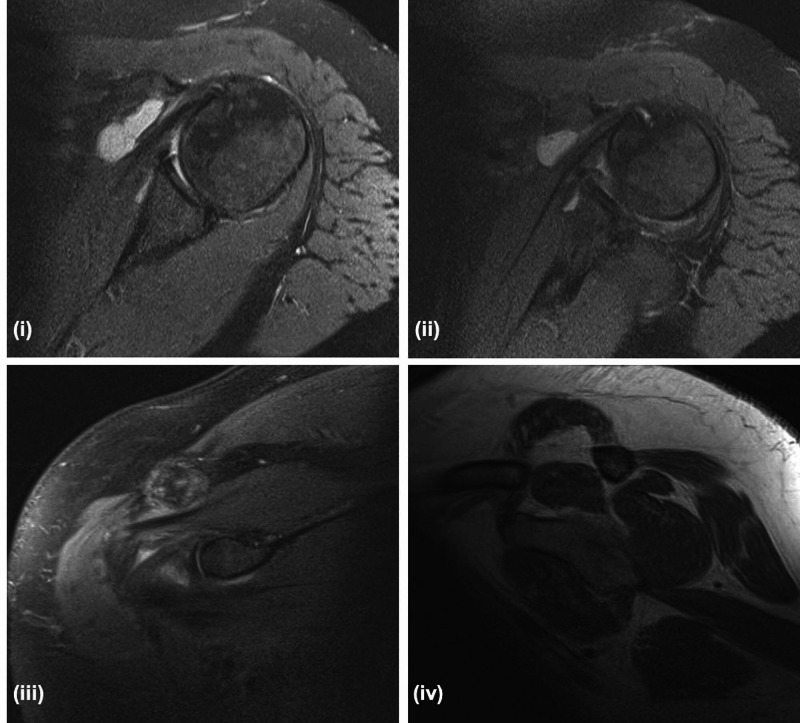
Magnetic resonance imaging (MRI) scan of the left shoulder (fat suppression-weighted) (i) axial image shows a residual subcoracoid effusion, but intact subscapularis tendon; (ii) axial image shows a residual subcoracoid effusion, and an intact subscapularis tendon and muscle belly; (iii) coronal image shows an intact subscapularis tendon and muscle belly; (iv) T1-weighted sagittal image shows a residual subcoracoid effusion and an intact subscapularis tendon and muscle belly
